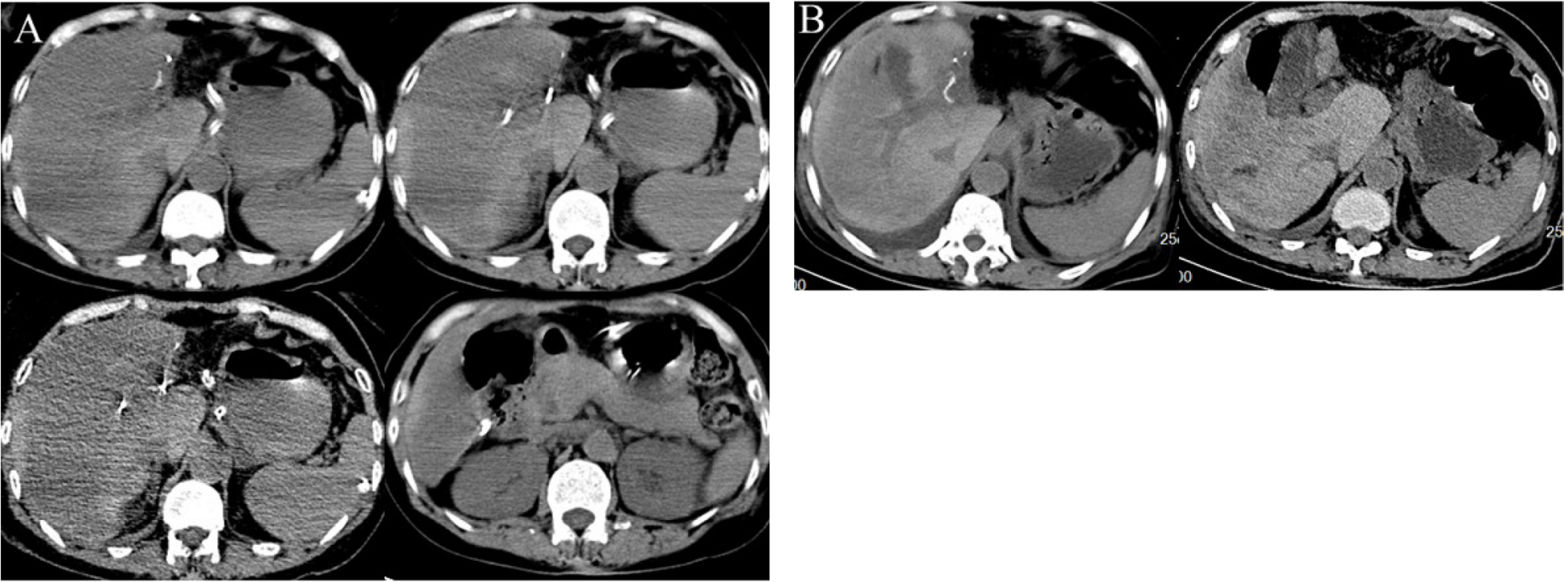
A Emergency abdominal CT showing marked liver atrophy and broadly heterogeneous hypoattenuating areas implied heterogeneous hepatic necrosis. B Fourteen days after the operation, follow-up CT claimed the disappearance of the heterogeneous hypoattenuating areas coupled with the recovery of the patient’s liver volume
